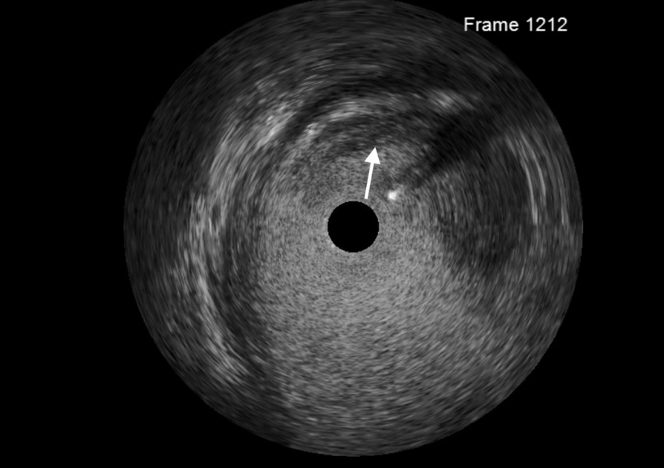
Intravascular Ultrasound of Distal Left Main Coronary Artery Intravascular ultrasound of the distal left main artery showing a lesion with mixed echoic and hypoechoic characteristics representing thrombus.
